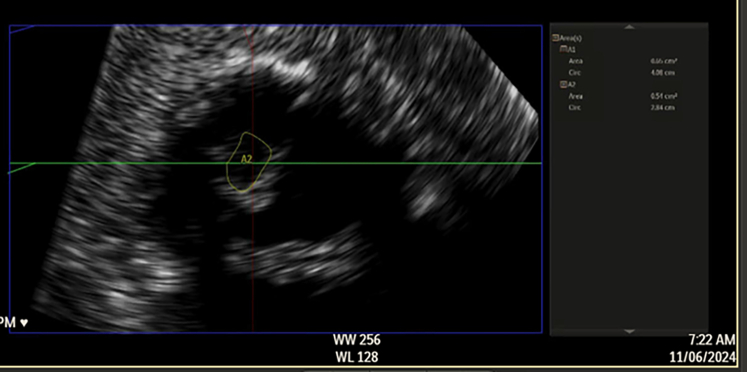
Transesophageal Echocardiography Showing Mitral Valve Area 0.51 cm^2^ by Planimetry
